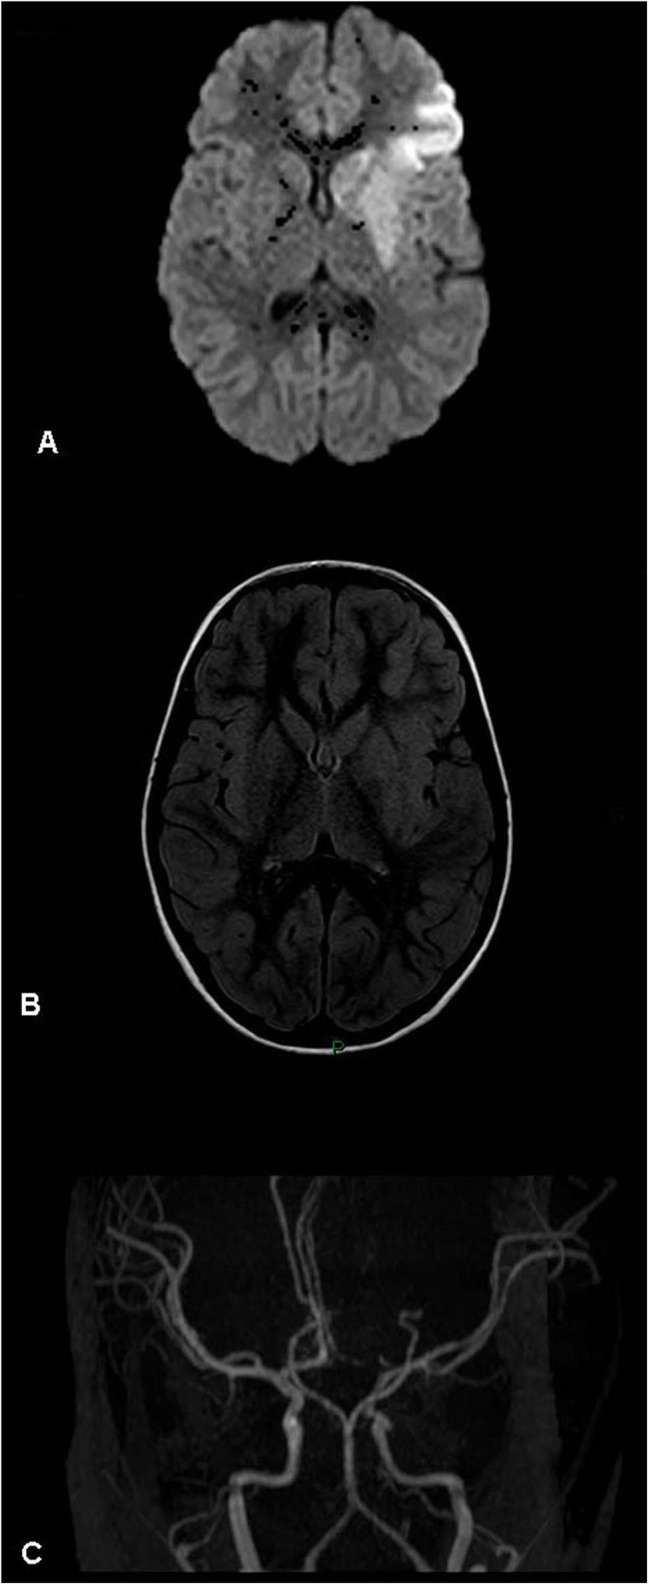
Acute left basal ganglia infarct – magnetic resonance (MR) findings (patient 10, Table 1). (A) Diffusion weighted image. (B) MR FLAIR. (C) MR angiogram (3D-TOF). Left basal ganglia and perisylvian acute stroke visualized mostly on diffusion weighted imaging. No mass effect. The angiogram shows an atretic left A1 segment (anatomic variant). No evidence of arterial occlusion. Left M2 segment focal narrowing is visualized.
